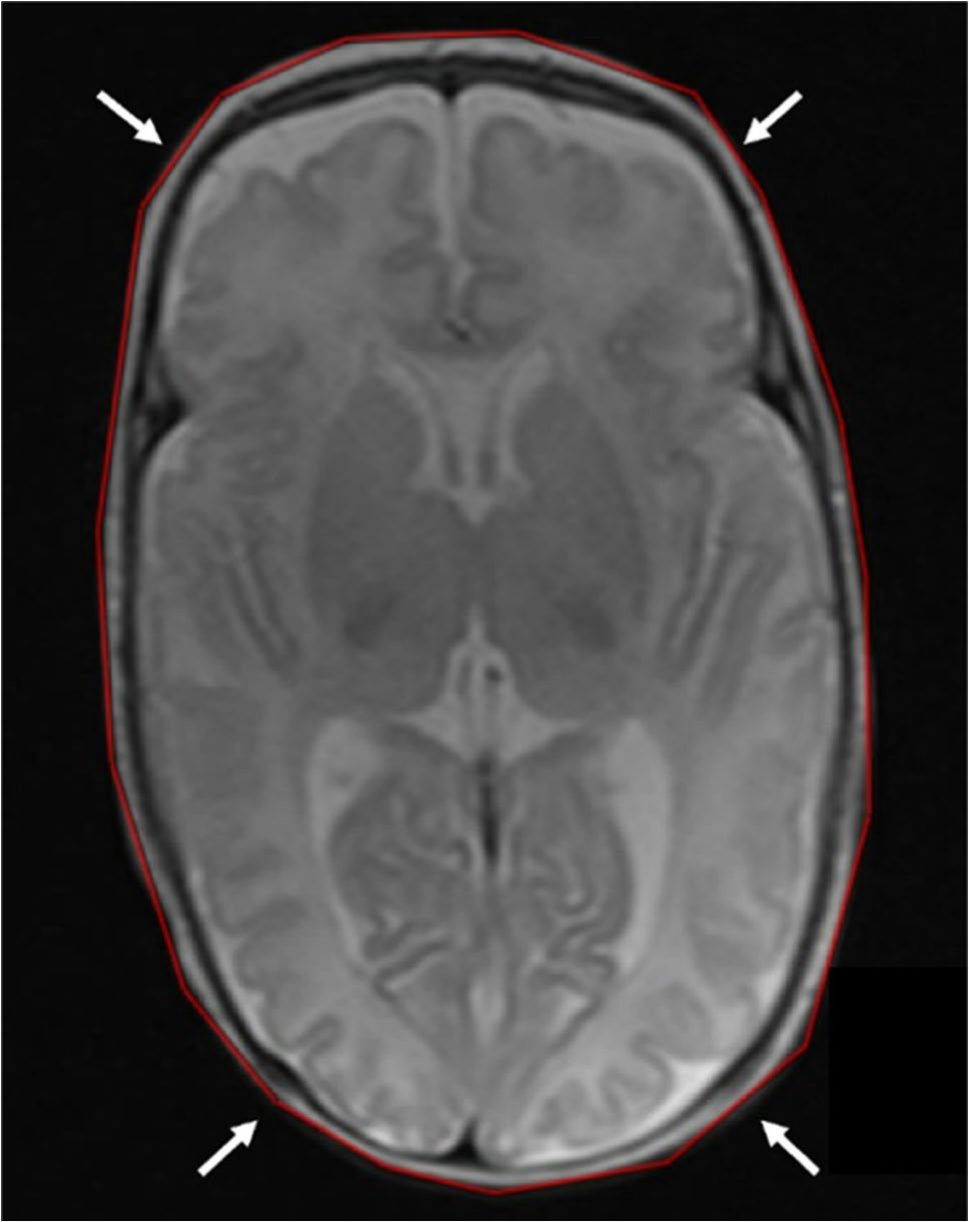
A 14-week-old girl born extremely premature at 27 gestational weeks with magnetic resonance imaging performed at term-equivalent age. Axial T2-weighted image without contrast demonstrates the measured head circumference, using multiplanar reconstructions, with measurement taken from the supraorbital bulge, encompassing the widest supra-auricular and occipital dimensions of the head (*arrows*). The infant had a left-sided germinal matrix-intraventricular haemorrhage grade 1 identified on cranial ultrasound performed at 7 days of age
